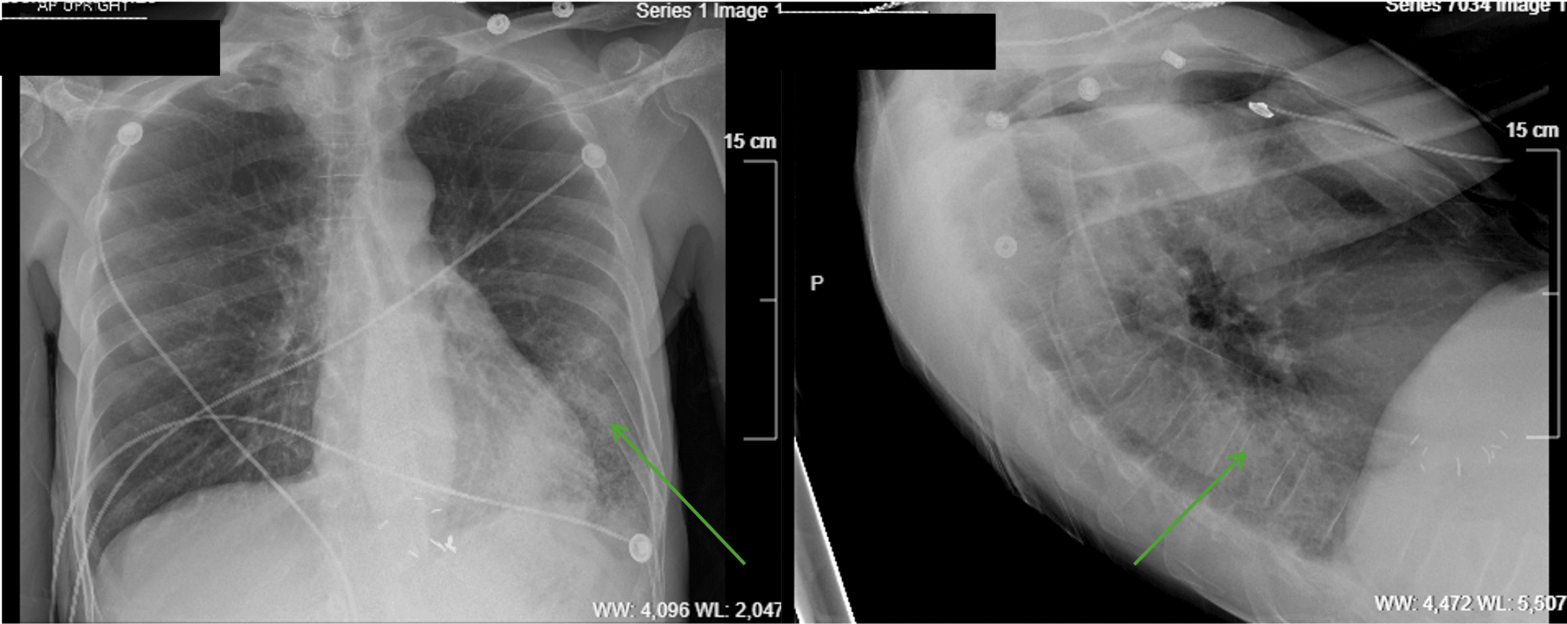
Two-view chest radiograph on day 0 exhibiting lower lobe acute process (green arrows).
